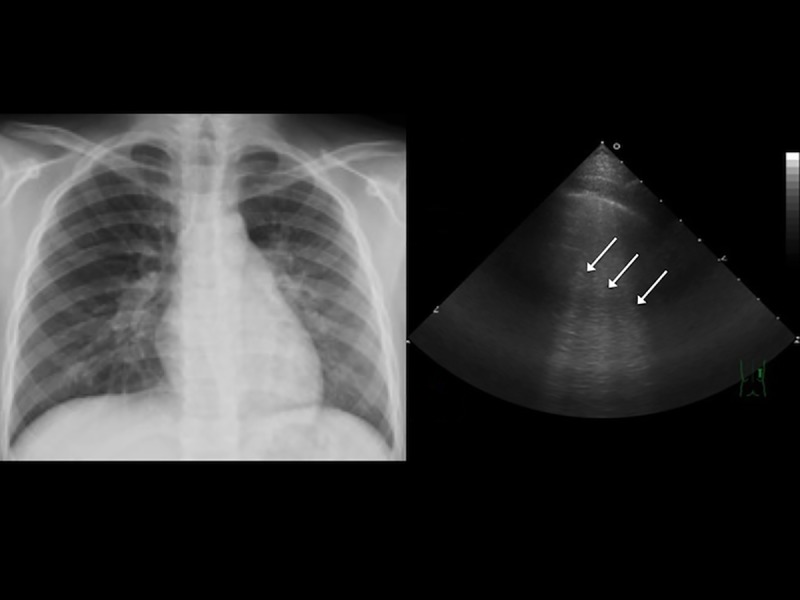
(A) Chest radiograph of a 12-year-old boy with left lower lung pneumonia. (B) Transthoracic ultrasonography revealed multiple B-lines (arrows) in the left anterior lower lung.
